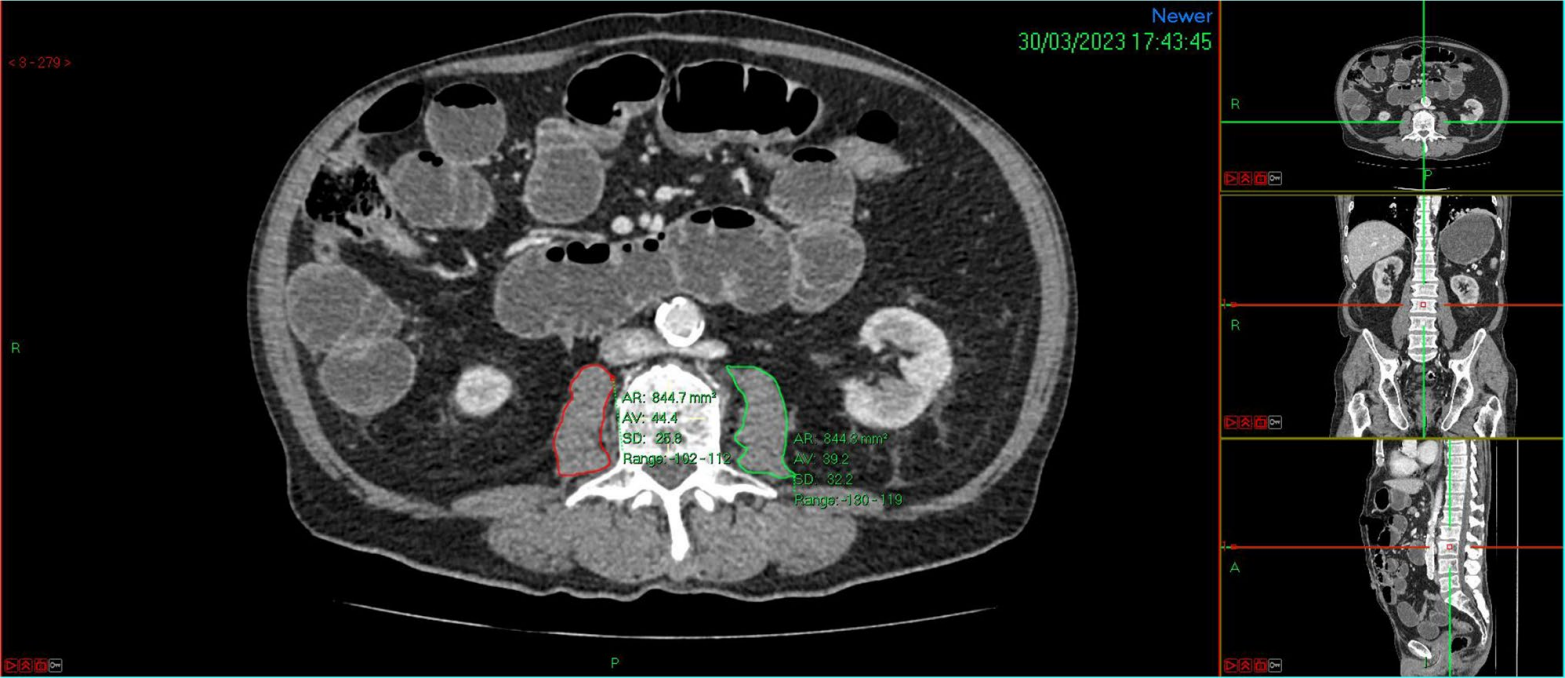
CT Abdomen and Pelvis, at the level of L3 vertebra, demonstrating method of measuring total psoas area (TPA). Outlines of left (green) and right (red) psoas major muscle were manually traced on PACS
